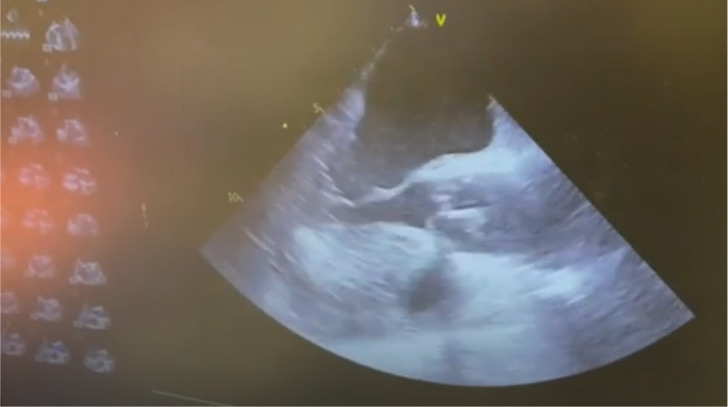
Final appearance of the reconstructed aortic valve on transesophageal
